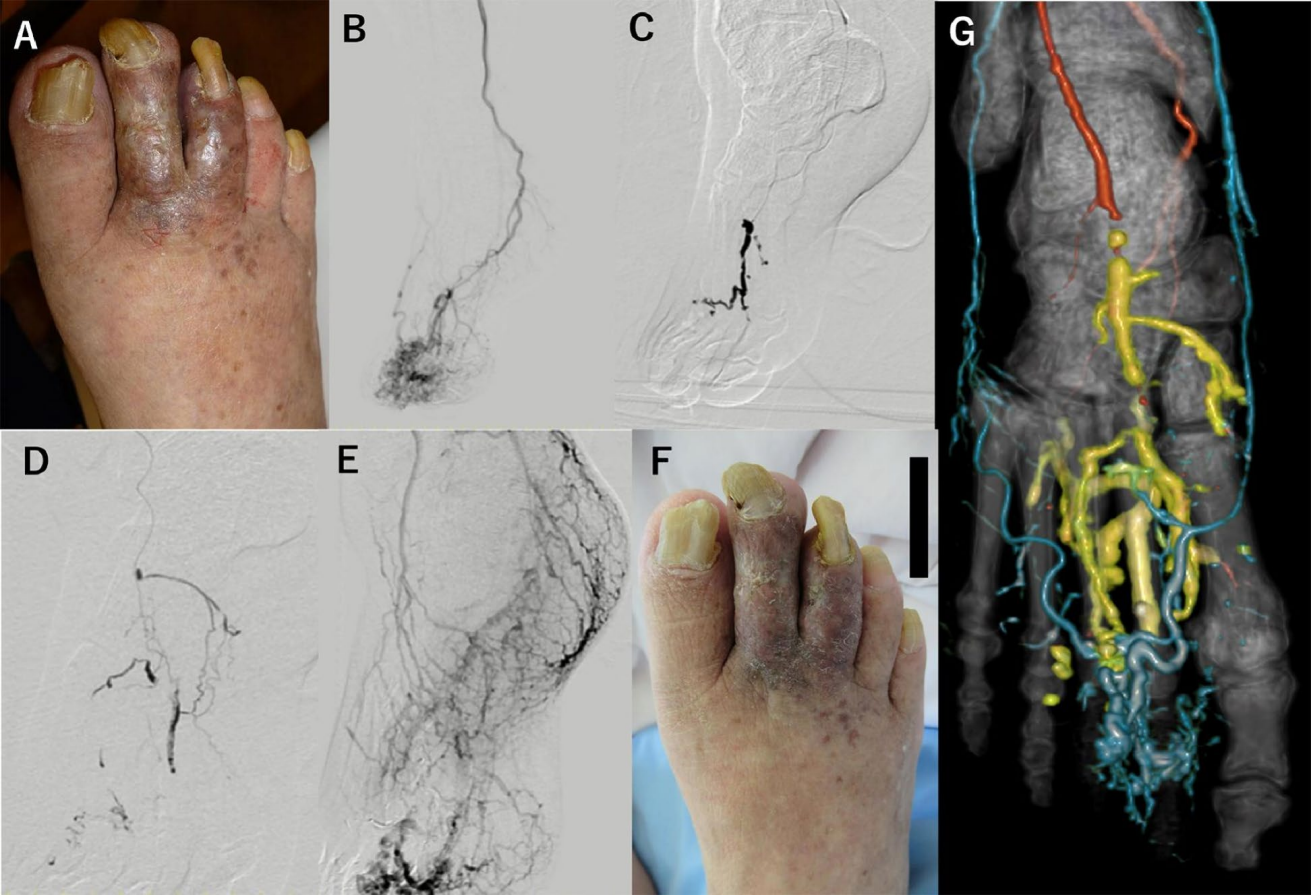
Right toe appearance and embolization findings. The second and third toes were infected (A). Digital subtraction angiography showed arteriovenous malformation (AVM) (B). Embolization using a mixture of *N*-butyl cyanoacrylate (NBCA) and lipiodol from the right plantar artery (C) and dorsalis pedis artery (D). Significant decrease in AVM perfusion after embolization (E). Infection of the toes was improved by antibiotic use and embolization (F). Computed tomography angiography after embolization showed decreased AVM flow compared to Fig. 1D. The embolic agent is indicated by the yellow segments (G)
